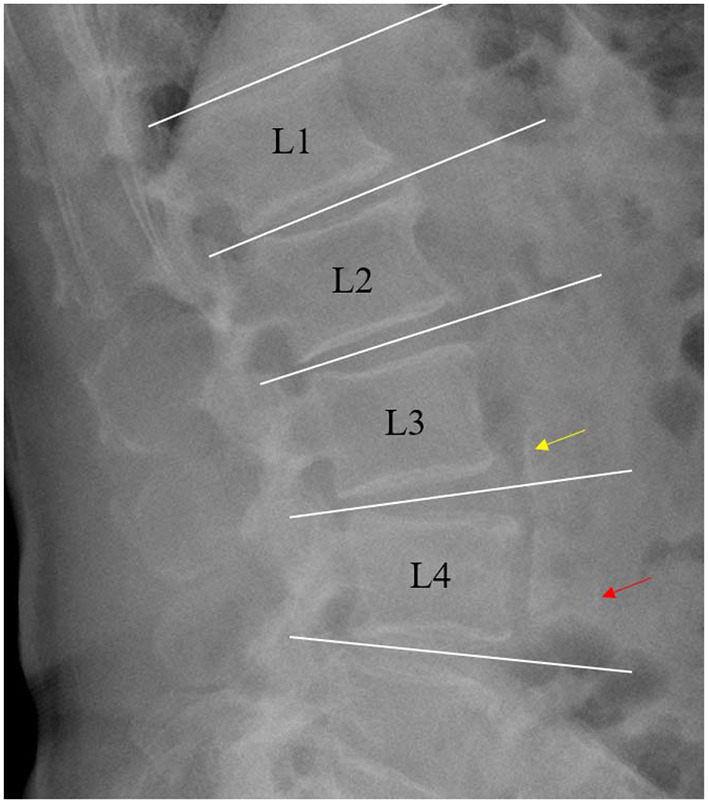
Example of lateral abdomen radiograph showing calcification of the anterior (red arrow) and posterior wall (yellow arrow) of the abdominal aorta.
